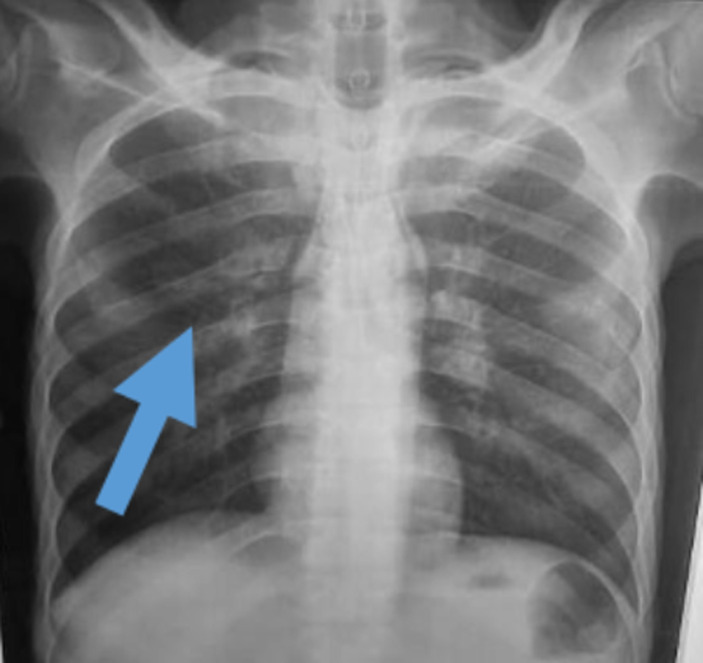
radiographie pulmonaire au bout de 6 semaines de traitement antituberculeux régression de la lésion cavitaire
